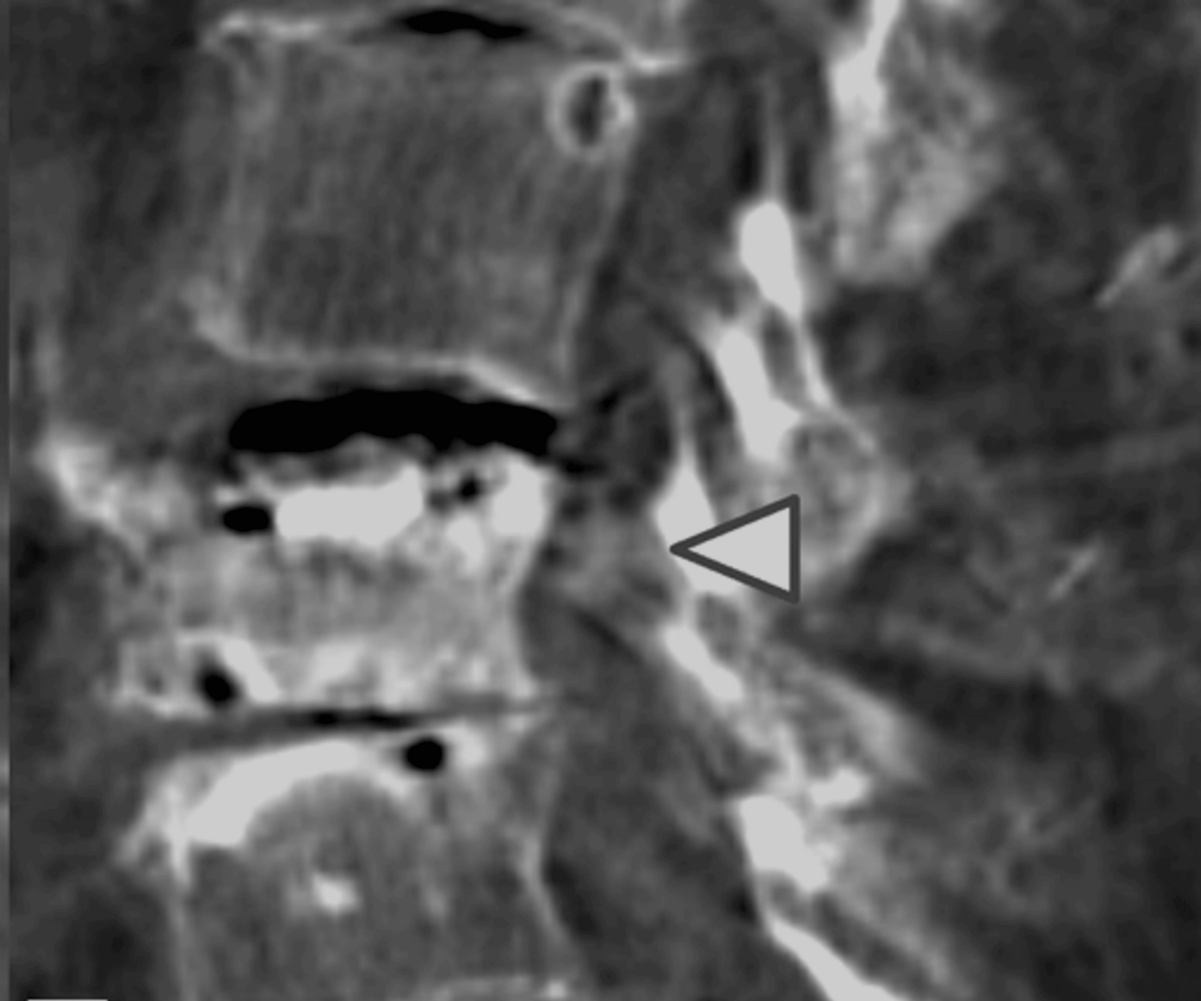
Sagittal view of intraoperative cone-beam 3D-CT Intraoperative cone-beam 3D-CT showed a reduction of the bone fragment (white arrowhead).
